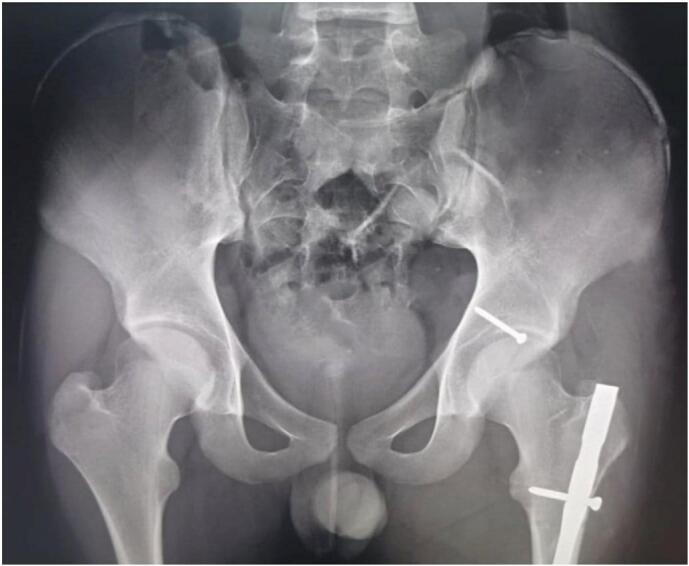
Radiographs at the last follow-up revealed no evidence of avascular necrosis of the femoral head.
